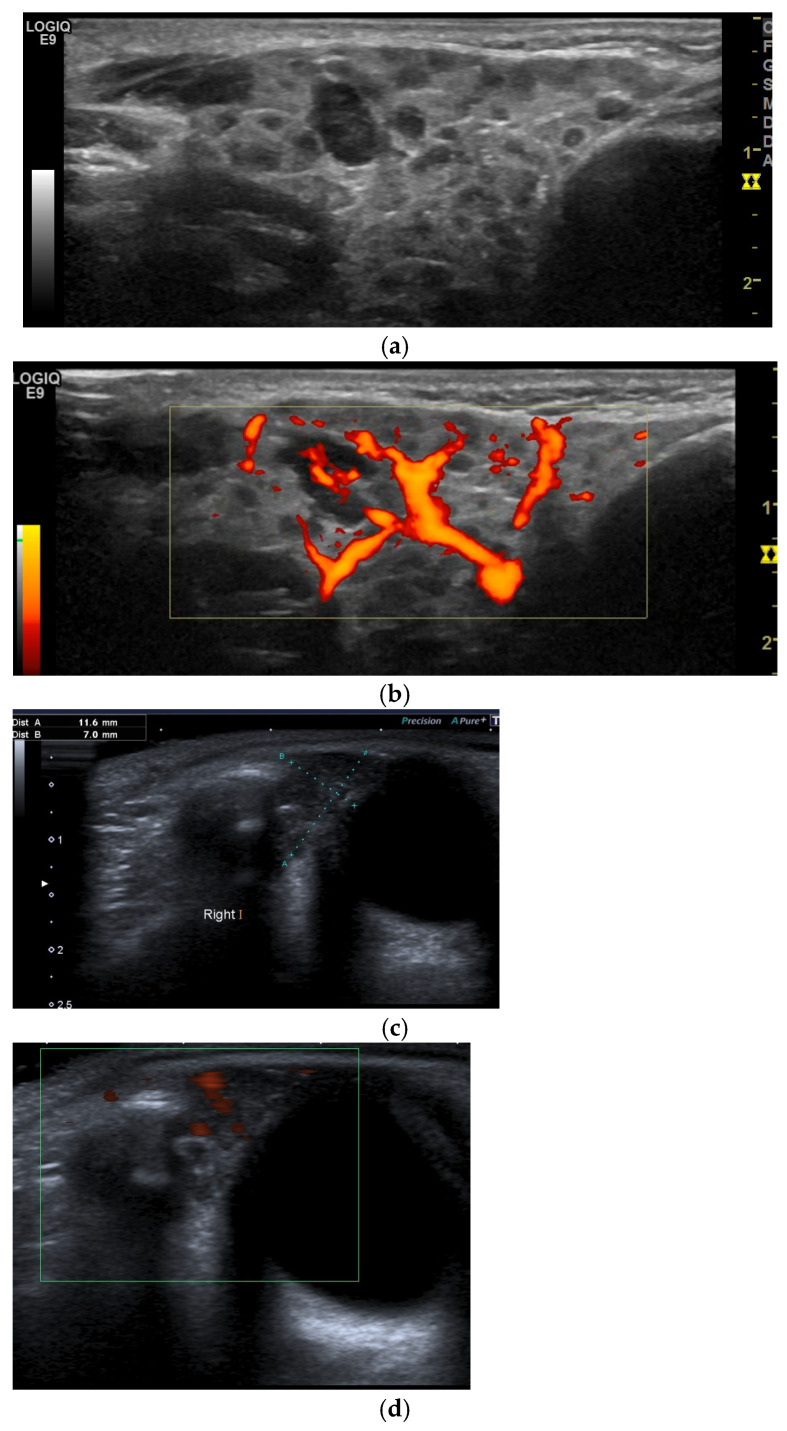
Ultrasound findings of the salivary and lacrimal glands in patient 1. Parotid gland ultrasound in B-mode (a) and colour Doppler (b) demonstrates an inhomogeneous echotexture with multiple hypoechoic areas, intraglandular lymph nodes, and increased parenchymal vascularity. Lacrimal gland assessment in B-mode (c) and Doppler (d) shows a normal ultrasonographic appearance.
